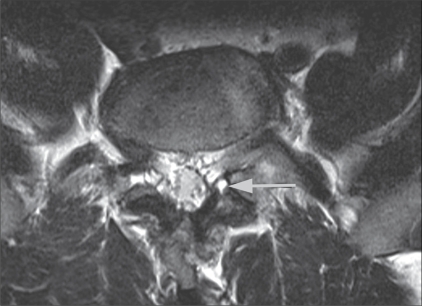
Axial T2W MRI image at L3–4 shows a left facet synovial cyst (arrow).
